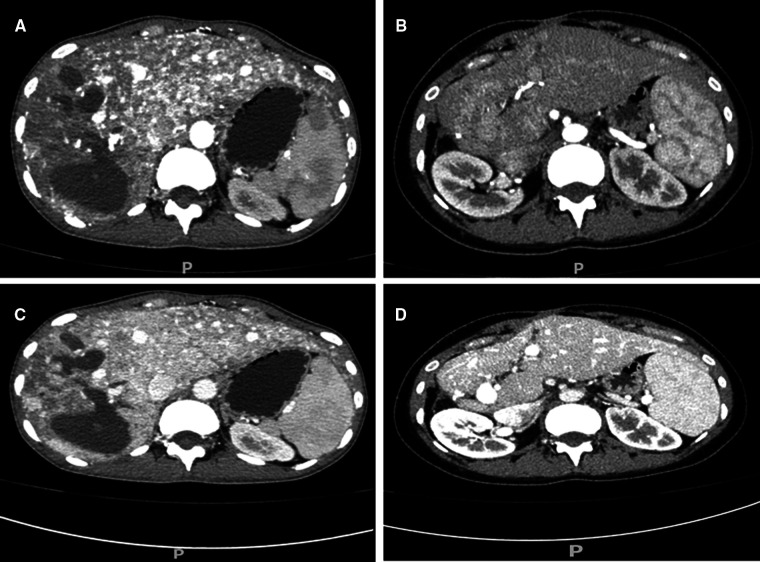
CT image comparison of the hepatic arteriovenous shunt before and after operation. CT arterial phase showed a significant decrease in telangiectasia and the shunt (A, preoperative; B, postoperative). Enhanced CT in the portal phase also showed a significant decrease in the shunt (C, preoperative; D, postoperative).
